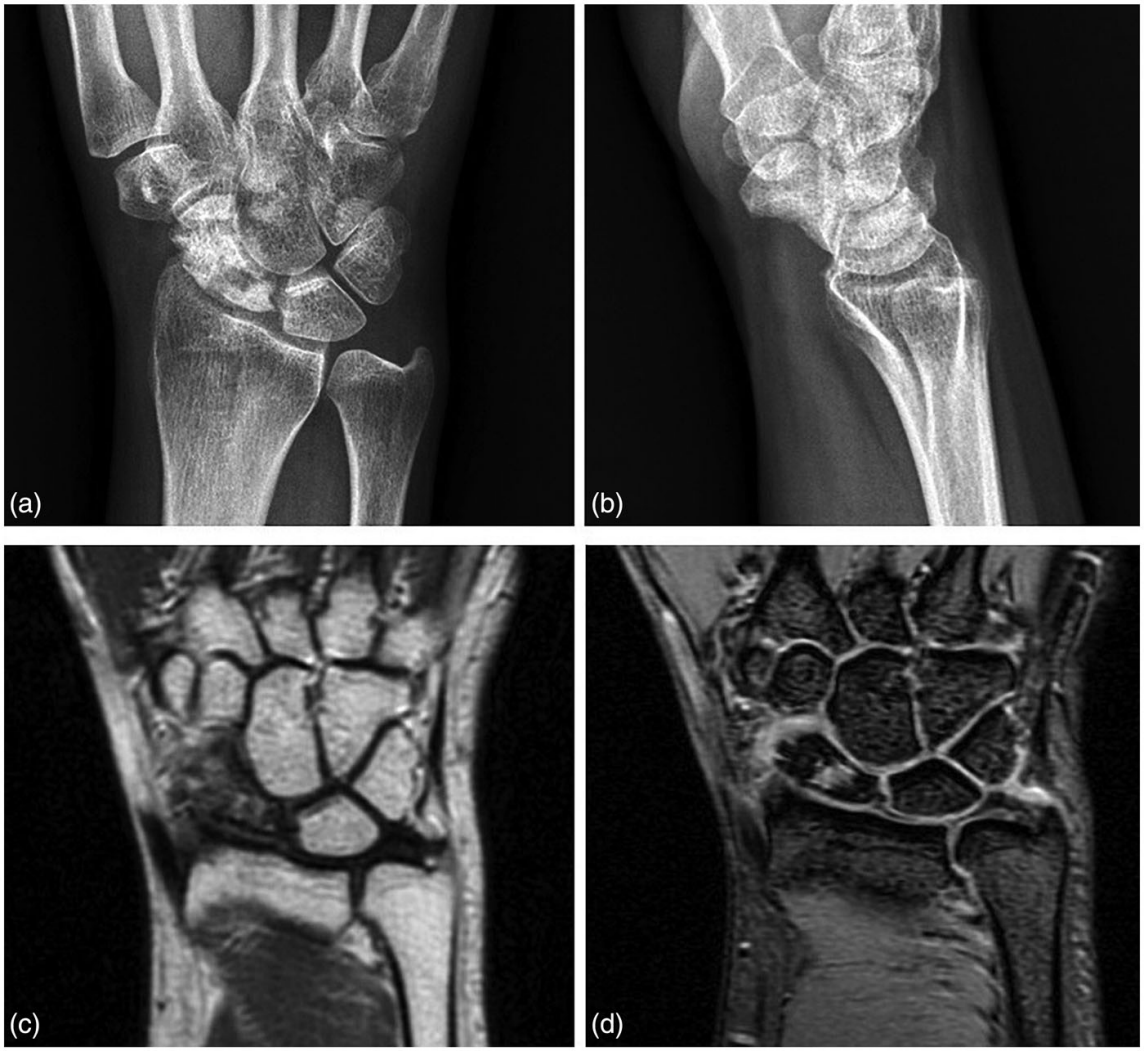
(a,b) Radiographs showing the right proximal scaphoid fracture nonunion with bony density change; (c) Magnetic resonance imaging shows ischemic change with a diffuse hypointense marrow signal in the scaphoid fragments and (d) a nonunion cavity.
